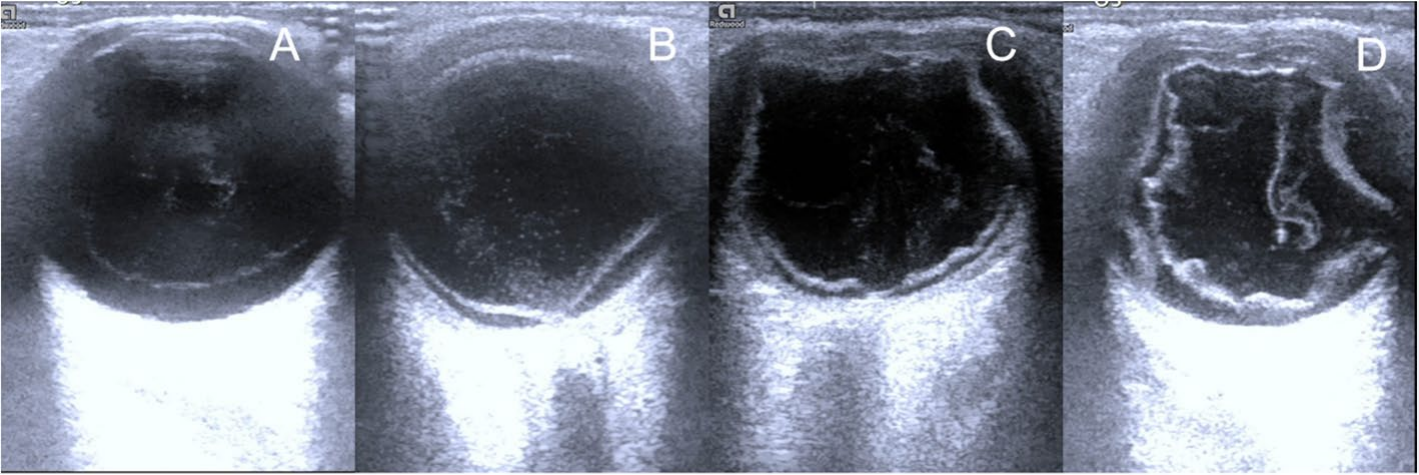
Ocular color ultrasonography of the left eye. A+B Day 3 post-injury: Retinal and choroidal detachment, indicating posterior segment involvement. C+D Day 30 post-injury: Marked globe atrophy with peripapillary hyperechoic lesions
